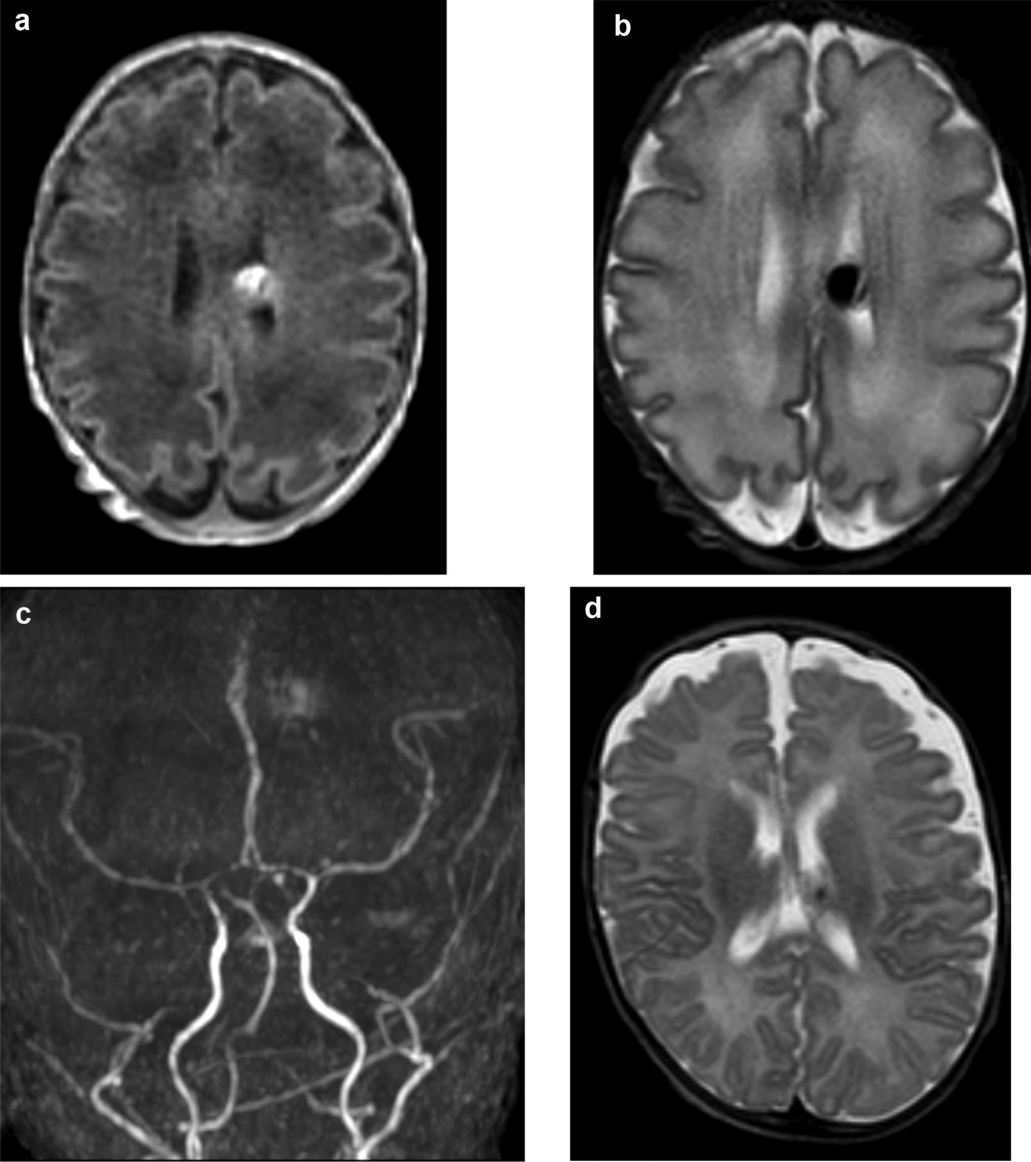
Follow-up brain MR scans: a, b T1- and T2-weighted images at 14 days showing complete thrombosis of the pouch. c A time-of-flight MR angiography shows disappearance of the vascular flow in the feeding vessels. d A further follow-up scan after 2 months demonstrates shrinking of the pouch
